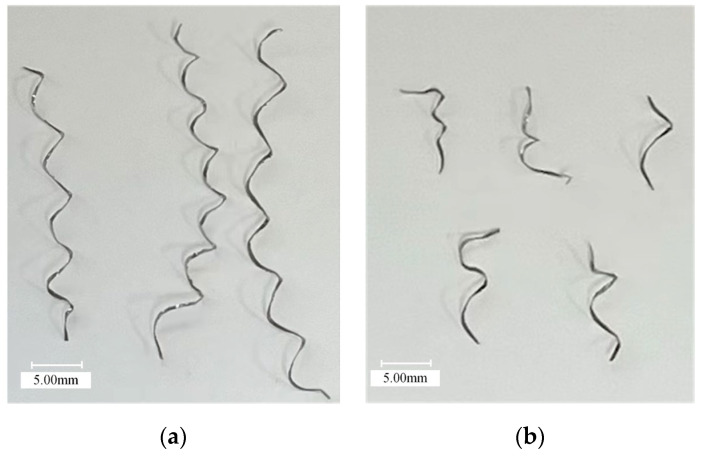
Chip morphology: (a) CT and (b) UAT.
